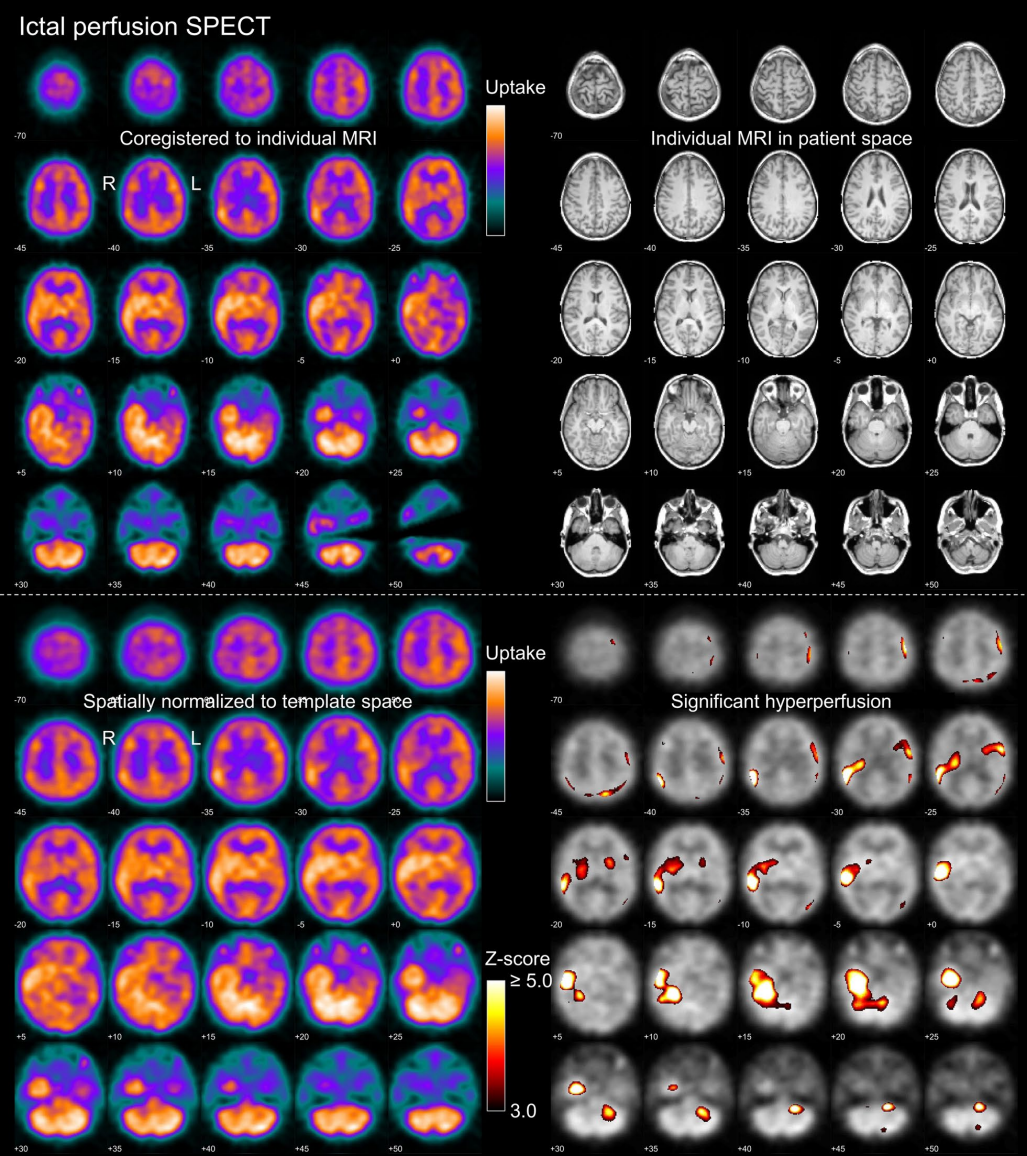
Standardized display for visual reading in the “ictal only” setting. The upper and lower parts were provided as page 1 and page 2 of a 2-page pdf-document. On the first page, the left side shows the patient’s ictal perfusion SPECT coregistered to the individual T1-weighted MRI shown on the right. On the second page, the left side shows the patient’s ictal perfusion SPECT after spatial normalization to MNI space, the right side shows the statistical hyperperfusion map thresholded at z ≥ 3.0 and overlaid to the patient’s ictal SPECT. The example images are from the same patient as in Fig. 1. Ictal SPECT was performed with 700 MBq ^99m^Tc-HMPAO injected 36 s after electrical seizure onset. The seizure continued for 70 s after the tracer injection
